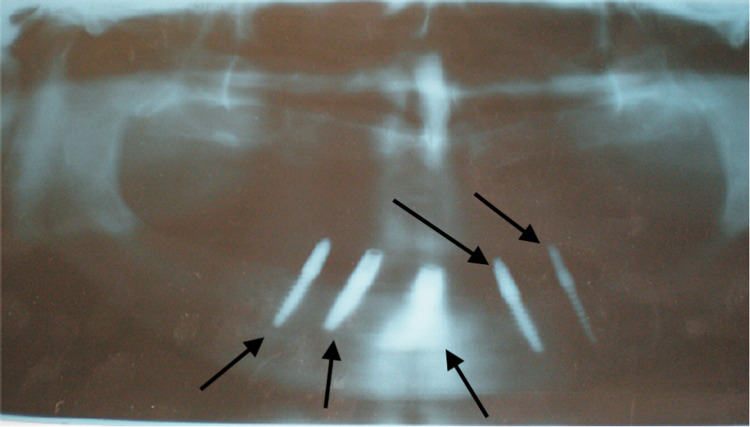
Orthopantomograph with Bi-cortical Screws and Implants
